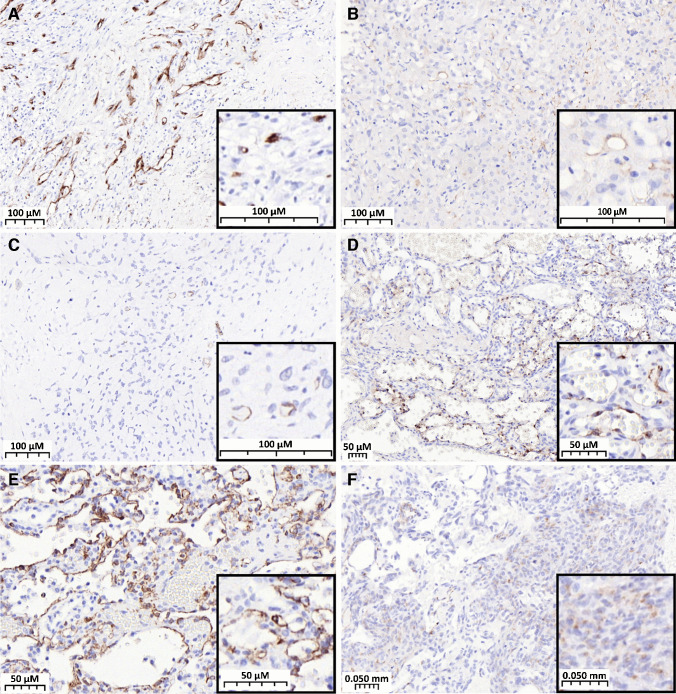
PSMA expression in vascular tumors. A Angiosarcoma of bone showing intense and diffuse expression of PSMA in the neovasculature (PSMA score 2), the tumor cells are negative. B Epithelioid hemangioma with PSMA index 1. C Epithelioid hemangioendothelioma with PSMA index 1. D hemangioma of the liver showing expression of PSMA in the endothelial cells forming the tumor (PSMA index 2). E Strong PSMA expression in the neoplastic endothelial cells in hemangioma of bone. F Weak expression (PSMA index 1) in the tumor cells of angiosarcoma of soft tissue
